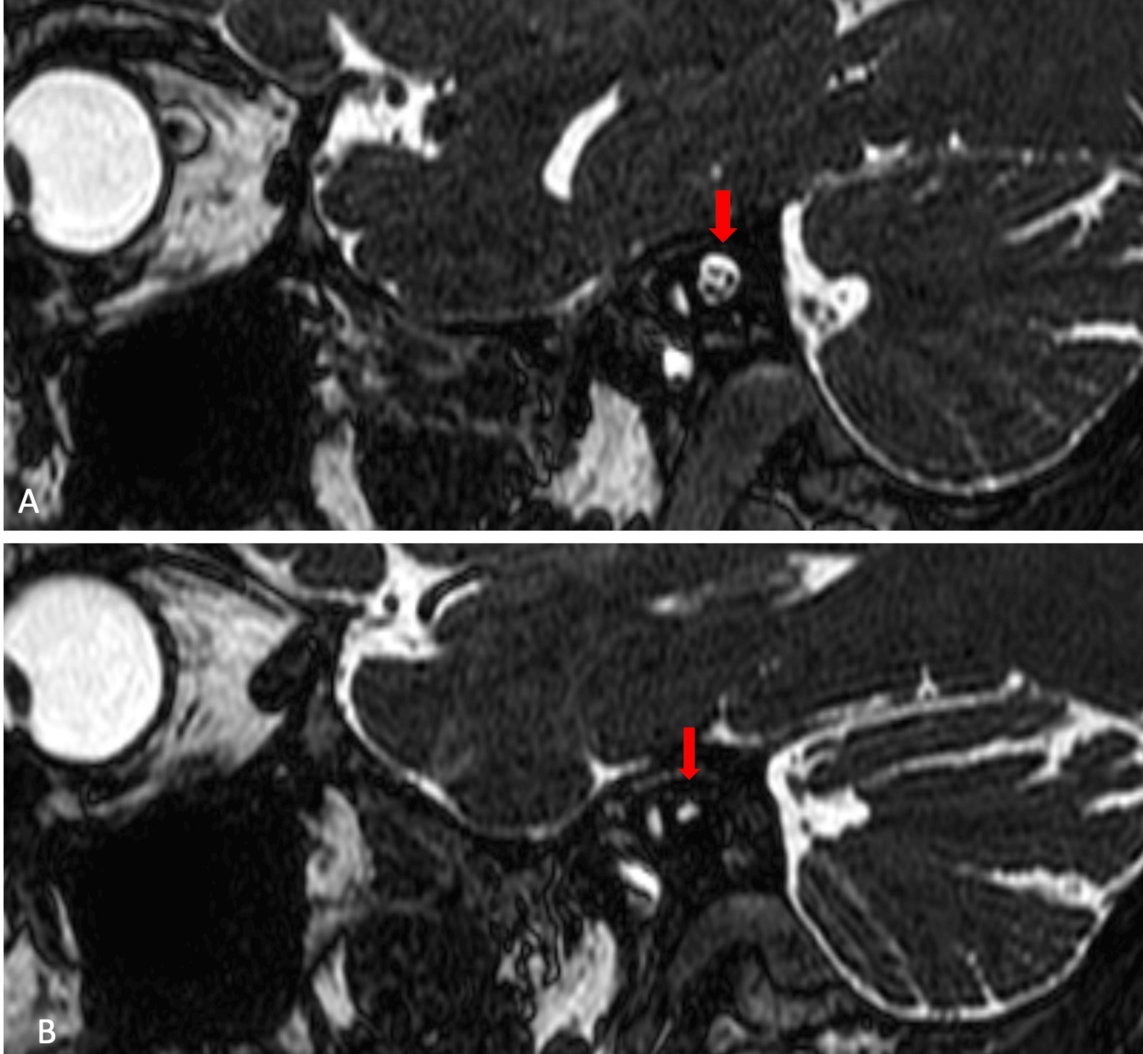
3D CISS sequence MRI in sagittal plane: (A) normal right IAC with common vestibular, cochlear, and facial nerves within and (B) hypoplastic left IAC with no identifiable neural component within 3D: three dimensional, CISS: constructive interference in steady state, MRI: magnetic resonance imaging, IAC: internal auditory canal
